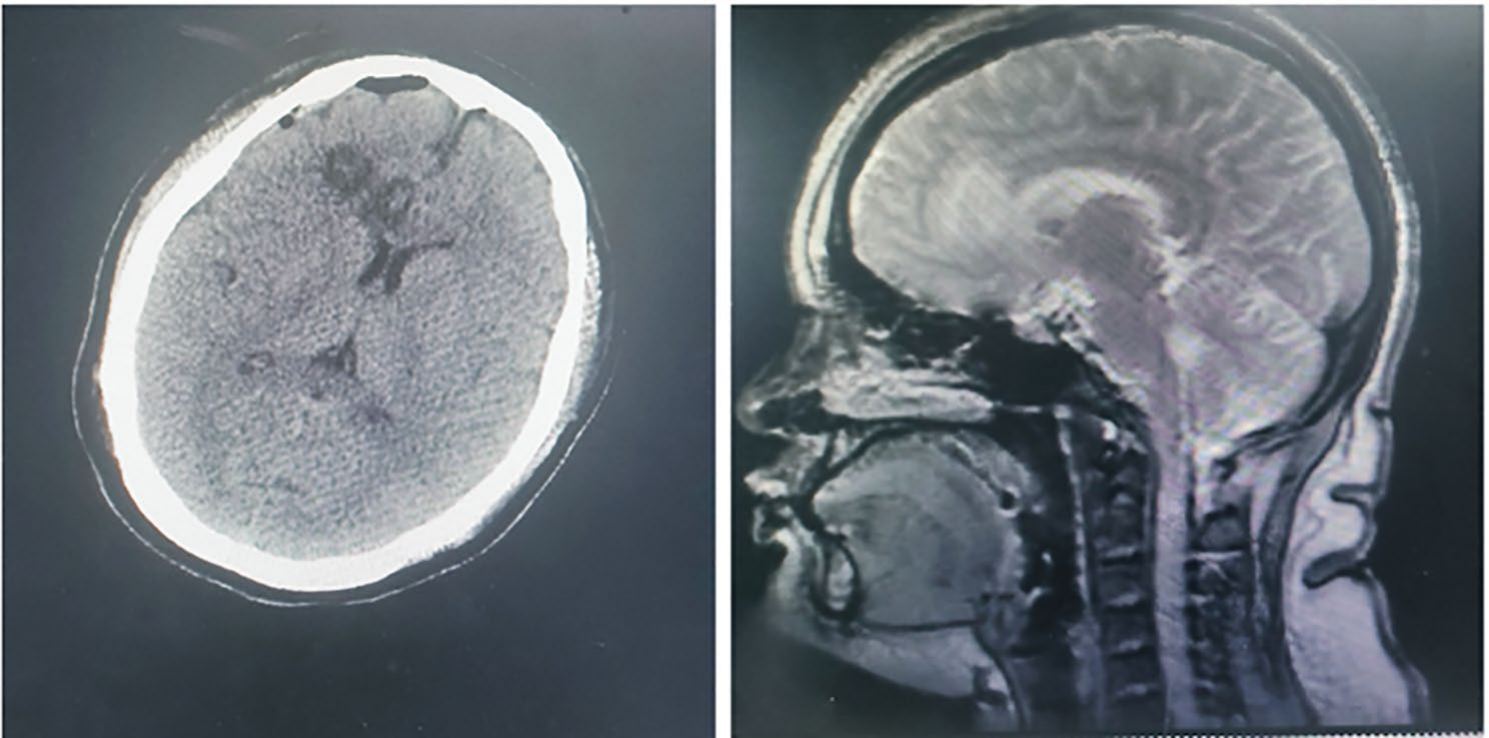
Postoperative cranial CT and MRI
